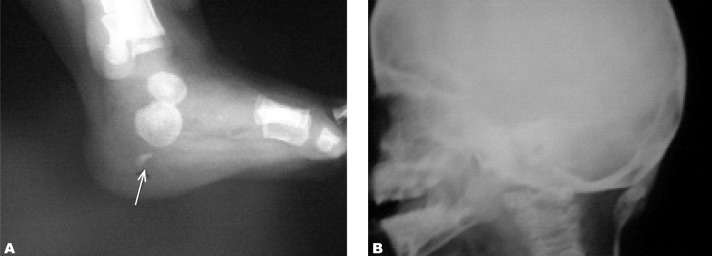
A. Foot and B. Neck radiography one year later at the age of two years show initiation of ossification in soft tissue masses
